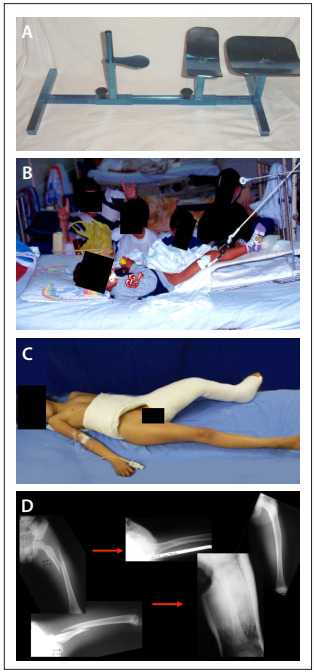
Children’s orthopedic table (A) for traction (B) and cast (C), for children with femoral shaft fractures; and radiographs showing the reduction control before and after the cast was applied (D).
